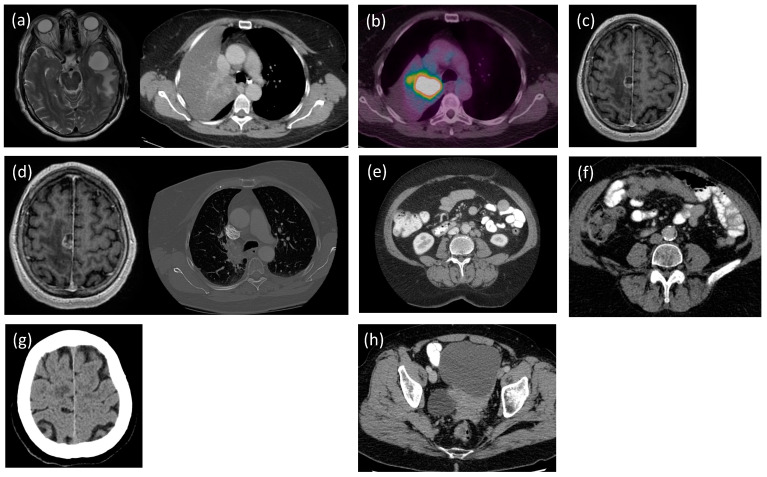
Patient scans. (a) The magnetic resonance imaging (MRI) of the head shows cystic metastasis (3.03 × 2.96 × 3.13 cm), and the computed tomography (CT) of the thorax shows the collapsed right upper lobe of the lung (8 March 2017). (b) Positron emission tomography (PET) of the whole body demonstrates FDG uptake in level 2 lymph nodes, palatine tonsils, and the tumor in the right upper lobe of the lung (27 April 2017). (c) MRI of the head shows the new solid and cystic enhancing mass (3 November 2017). (d) CT of the head shows tumor progression (1.6 × 1.5 × 1.9 cm), and CT of the thorax shows central tumor progression (9 March 2018). (e) CTs of the abdomen and pelvis show a new liver lesion and increased pelvic lymphadenopathy (10 July 2019). (f) CT of the abdomen shows new peritoneal deposits (10 × 3.4 cm) (24 March 2022). (g) CT of the head suggests brain lesion progression (8 December 2022). (h) CT of the abdomen shows worsening of the ovarian lesion (8.5 × 9.8 cm) and the metastatic deposits in the liver (5 mm) (29 March 2023).
